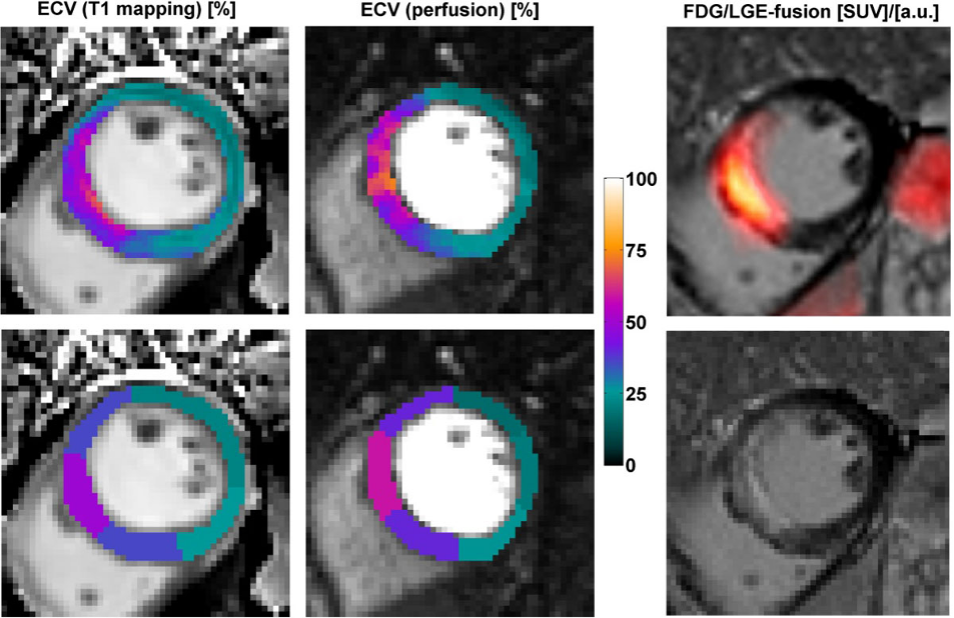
Example slice showing pixel wise and sector wise evaluation of ECV from T1 mapping and perfusion imaging in the left and center columns. The upper right image shows ^18^FDG uptake, the lower right image shows consistency with Late Gadolinium Enhancement
